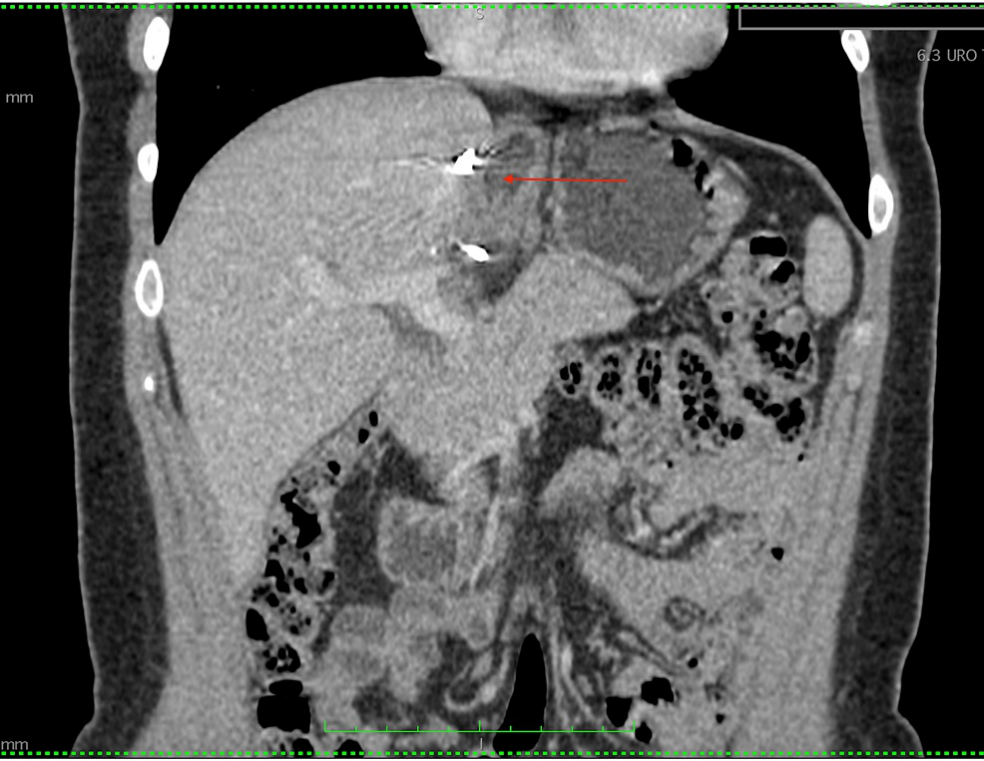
Follow-up CT scan five years after surgery. Following resection, the postoperative anatomical location (red arrow).
